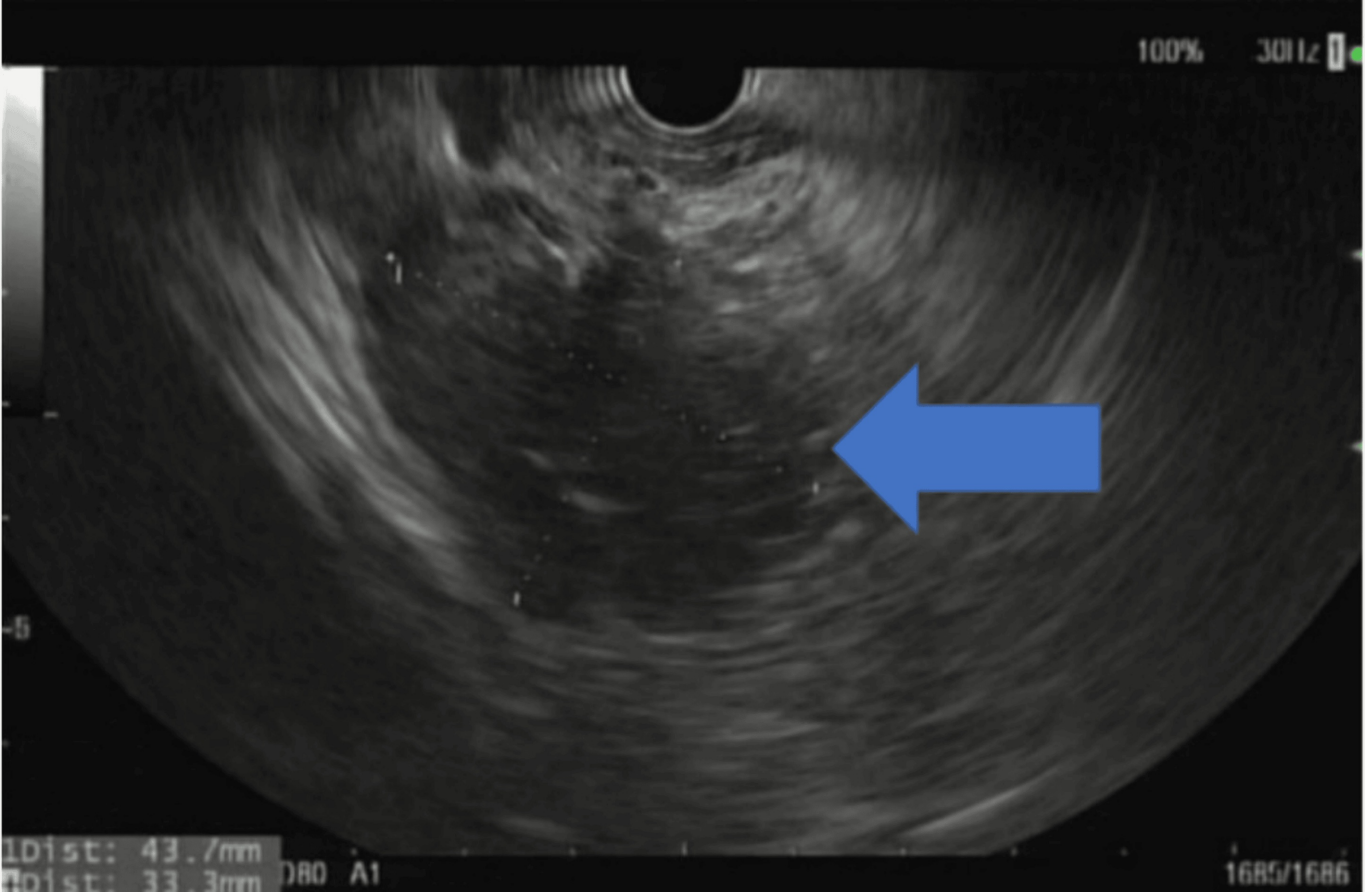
Endoscopic ultrasound showing an oval, hypoechoic, homogenous and solid shaped mass in the pancreatic head measuring 33 mm x 43 mm in maximal cross-sectional diameter (blue arrow).
